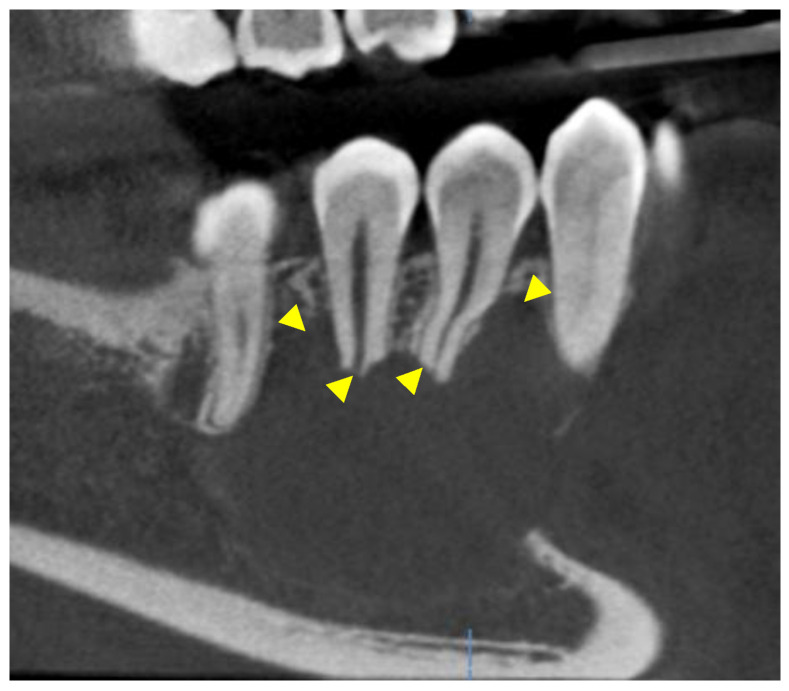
Oblique sagittal views on a CBCT study. The yellow arrowheads show evidence of root resorption and scalloping between the roots of the involved teeth.
